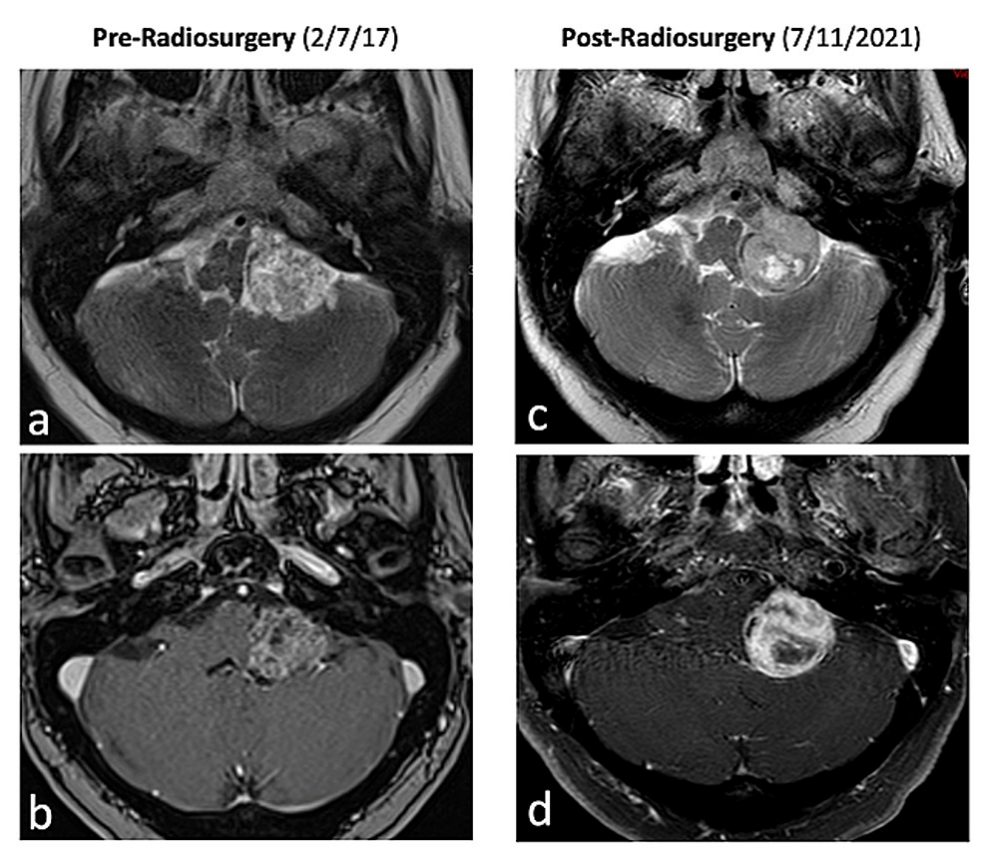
Original MRI brain imaging before and after radiation treatment Original brain imaging before (a-b) and after (c-d) radiation treatment is shown. (a) Axial T2-weighted image demonstrates a posterior fossa mass centered in the left cerebellopontine angle cistern and lateral medullary cistern with heterogenous T2 hyperintense signal. (b) Axial T1 post-contrast weighted image demonstrates heterogeneous enhancement. (c) Axial T2-weighted image demonstrates mild interval growth of the mass that shows an area of central T2 hyperintense signal due to cystic/necrotic changes. (d) Axial T1 post-contrast image demonstrates more prominent solid peripheral enhancement with central non-enhancement due to cystic/necrotic changes MRI: magnetic resonance imaging
